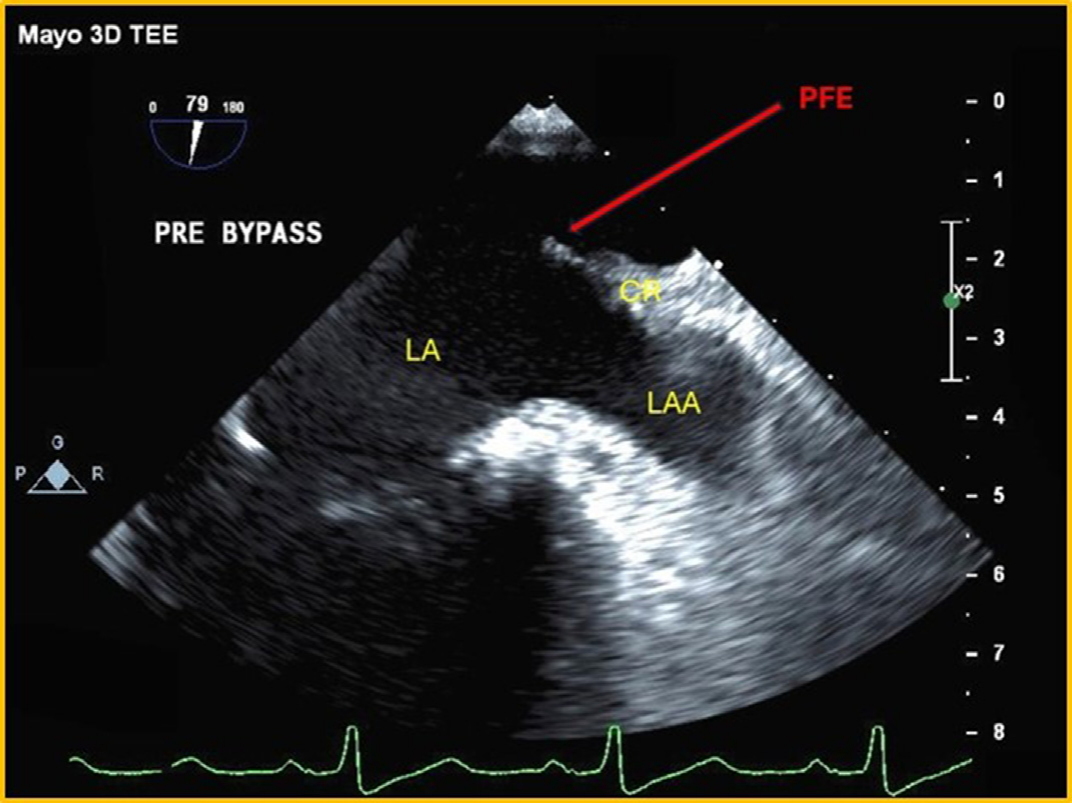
Transesophageal Echo Intraoperative 2-D TEE, focus on LAA: mass attached to the PVR. Abbreviations as in Figures 1 and 2.
